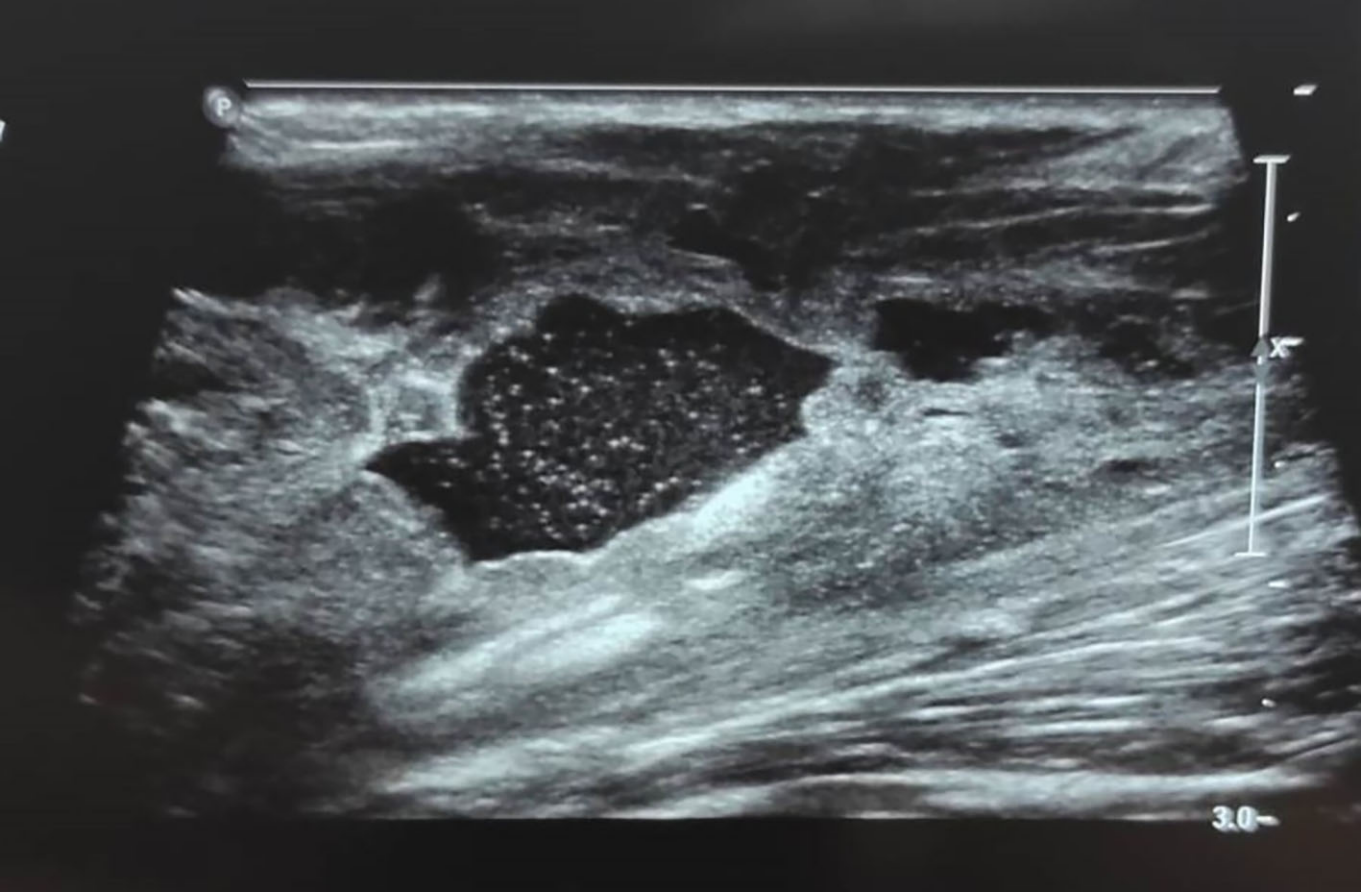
Utrasonography of the right knee reveals an echogenic collection of the right popliteal fossa.
